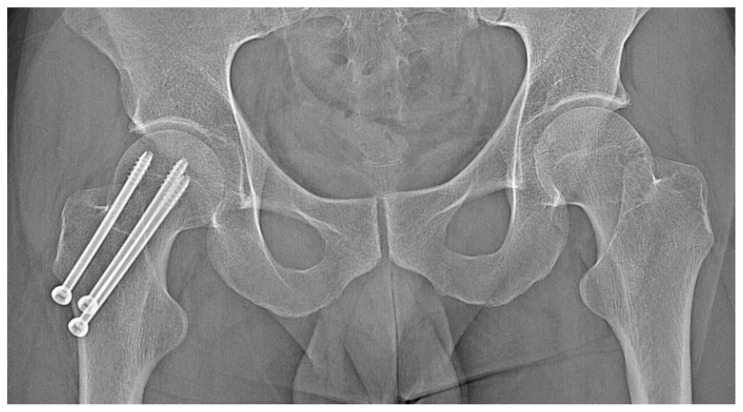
X-ray view of internal stabilisation with three cannulated screws of the right femoral neck.
